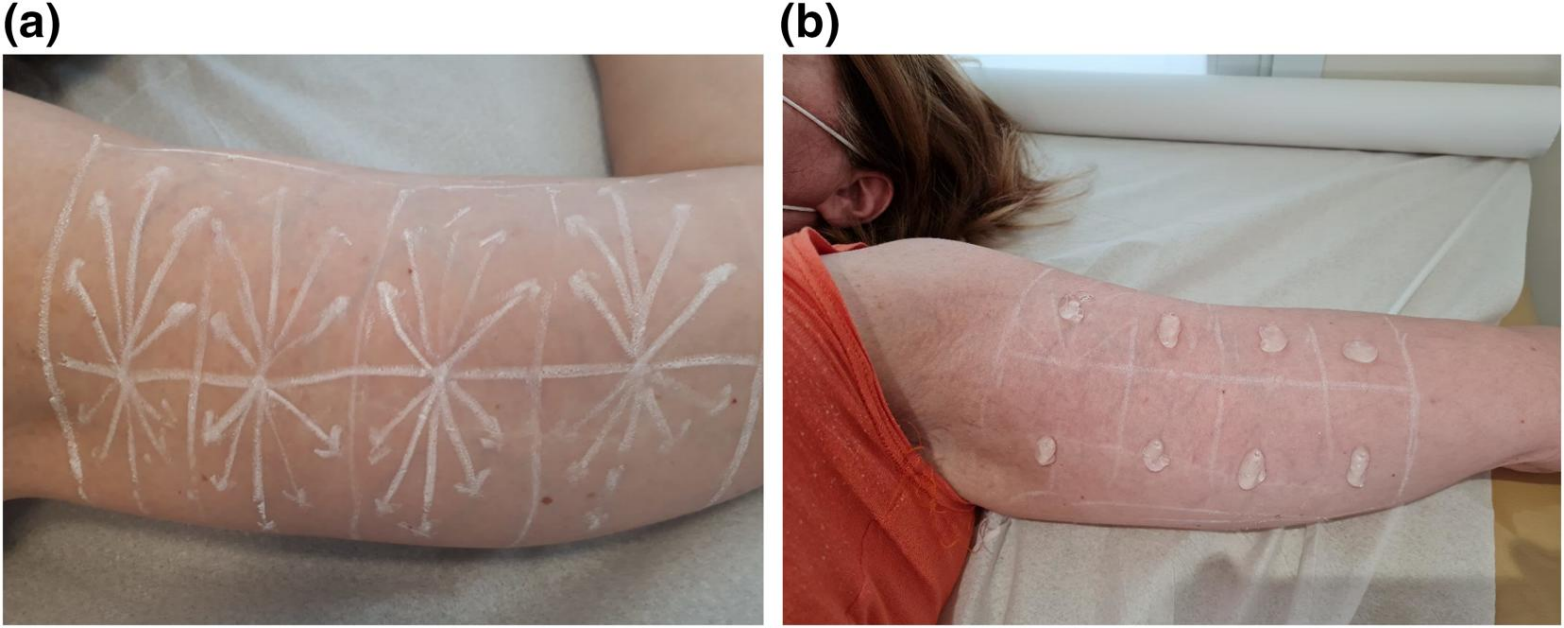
(a) Injection markings; (b) sites analyzed by high resolution ultrasound
